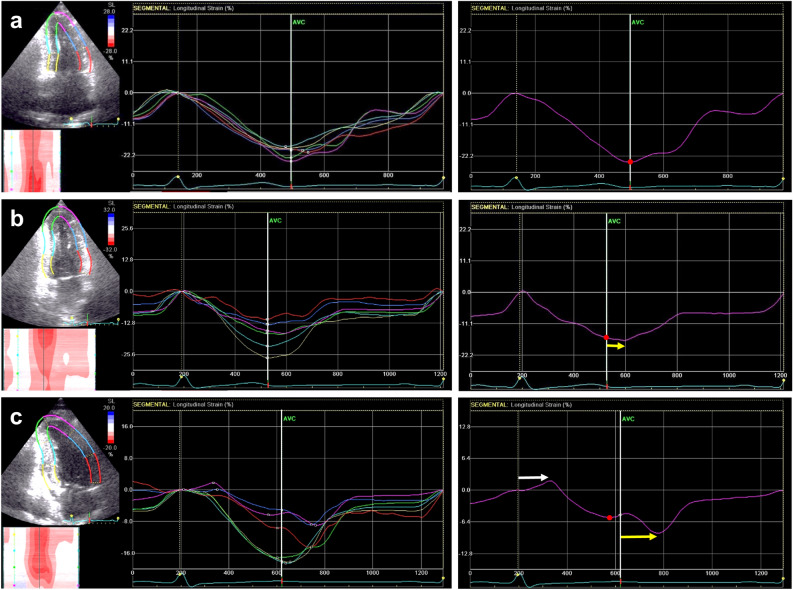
Representative strain–time curves in six segments (left) and the apical anterior segment (right) of the apical 2-chamber view. (a) An 83-year-old female with 29% diameter stenosis of the left anterior descending artery (LAD) derived from quantitative coronary angiography. (b) A 67-year-old male with 58% LAD stenosis. (c) A 56-year-old male with 88% LAD stenosis. The absolute value of the peak systolic strain (red circle) decreased, and early systolic lengthening (ESL) and postsystolic shortening (PSS) became larger with the severity of LAD stenosis, resulting in prolonged T_ESL_ (white arrow) and T_PSS_ (yellow arrow).
